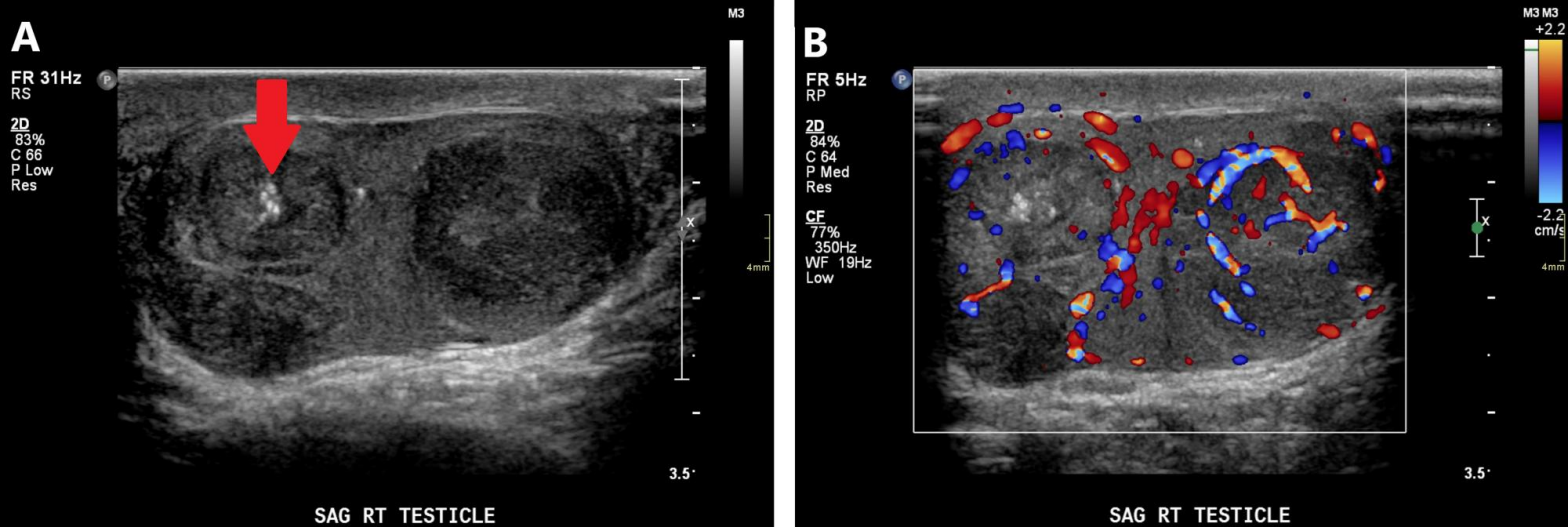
Nonseminomatous germ cell tumor. (A) Within the right testis, there are at least 3 heterogeneous predominantly hypoechoic lesions with irregular borders. One of the lesions contains central hyperechoic foci (red arrow) potentially representing hemorrhage, calcification, or fibrosis. (B) Doppler interrogation demonstrates vascularity indicating that these are not cystic lesions.
